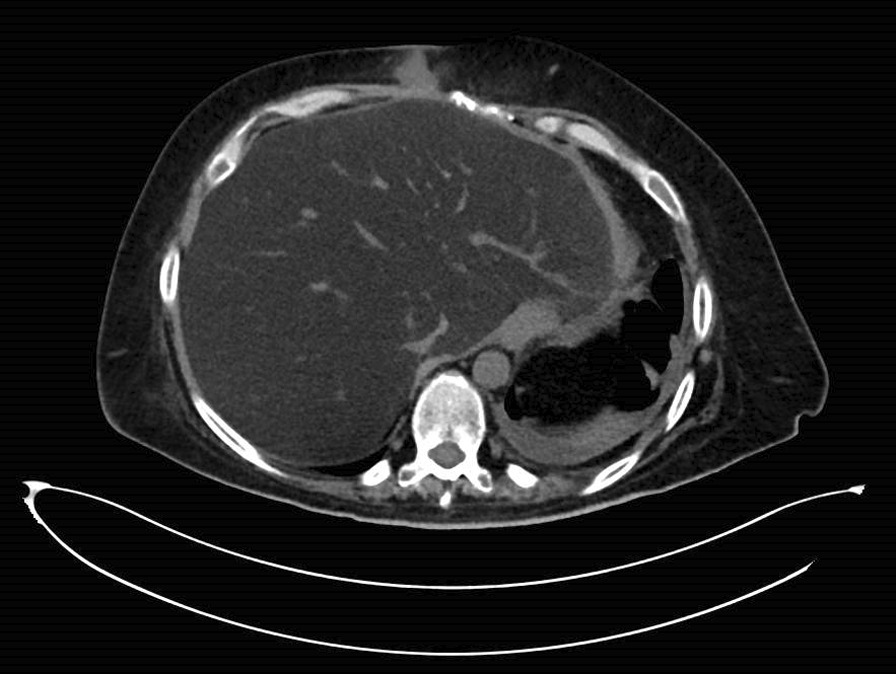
Radiological image. A computed tomography section of the patient, showing diffuse fatty infiltrates in the liver and a small pleural effusion on the left, which was unchanged with respect to previous imaging studies. There is a small surgical site infiltration in the abdominal wall
